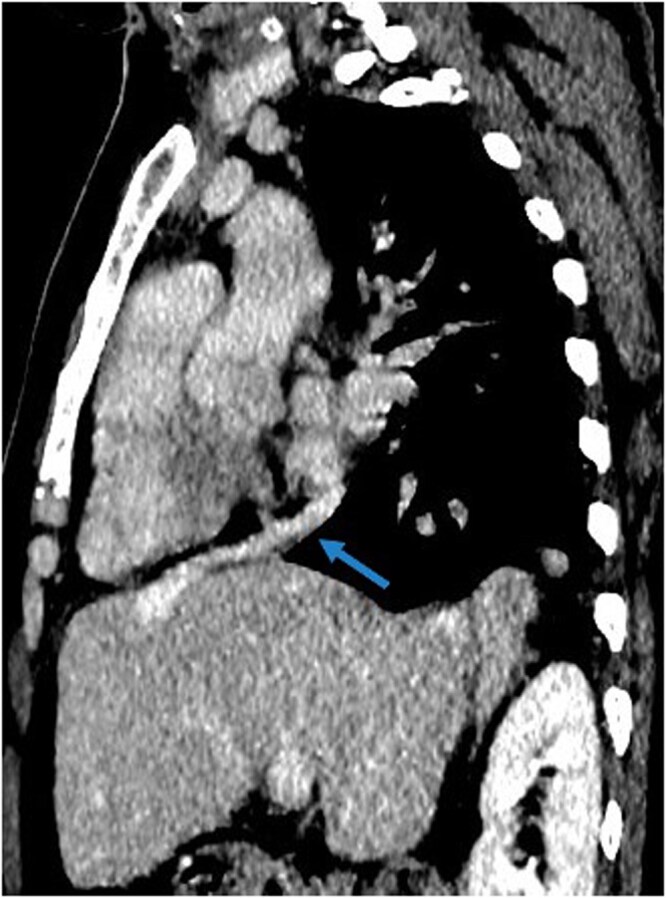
The sagittal CT image shows the venous duct originating from the hepatic veins, reaching the right atrium (arrow).
